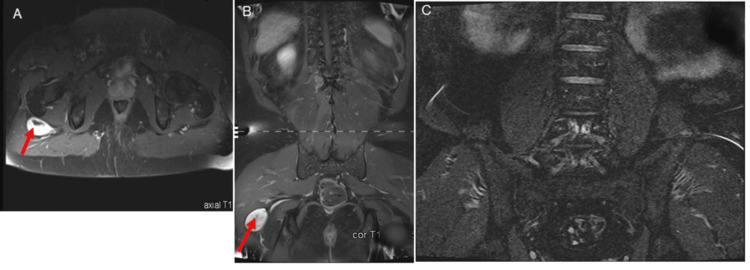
Preoperative and postoperative MRI images of the sciatic schwannoma. (A, B) Coronal and axial T1-weighted MRI of the lumbar spine and proximal thigh region with contrast, demonstrating a well-defined mass along the course of the right sciatic nerve, located posterior to the greater trochanter and ventral to the gluteus maximus muscle. The lesion measures approximately 4.7 × 2.4 × 5 cm (red arrow). (C) Postoperative MRI using an STIR sequence of the same region demonstrating no residual or recurrent mass, with expected postoperative changes in the soft tissues and surrounding musculature after gross total resection of the schwannoma.
